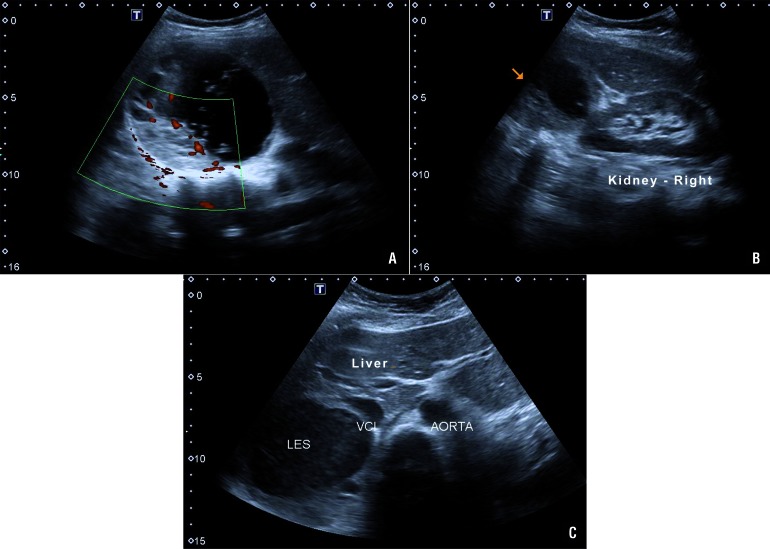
A) Ultrasound showing right adrenal tumor with more than 10 centimeters, with mixed cystic-solid components and doppler imaging confirm the presence of vascular flow; B) Arrow indicating the great mass between the liver (Liver) and the right kidney (Kidney - right); C) pheochromocytoma (LES) by compressing and pushing the inferior vena cava (VCI).
